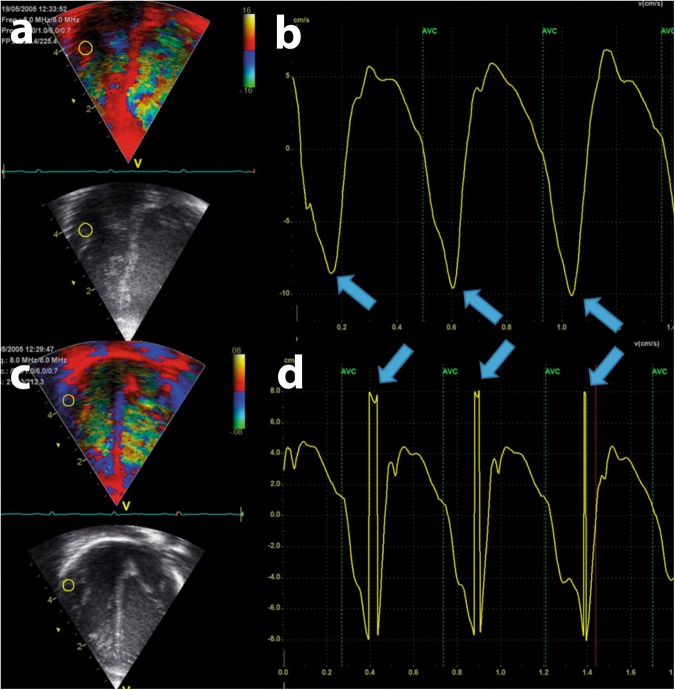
Example of non-aliased and aliased velocity measurements. Both panels show a color tissue Doppler velocity measurements of the right lateral atrioventricular plane in three consecutive heartbeats in a term neonate. The left part of both panels a and c show an apical four-chamber image with a sample area (yellow circle) of 3 × 3 mm. The right panels b and d show the velocity curves. The velocity scale is ±0.16 m/s in the upper panel and ±0.08 m/s in the lower panel. The negative peak velocity in diastole is approximately −0.09 m/s (blue arrows), within the velocity range in the upper panel but outside the velocity range in the lower panel. The ultrasound system therefore shows the velocity correctly in the upper panel and erroneously as a positive velocity close to the upper velocity limit in the lower panel. Note that there is complete fusion of diastolic velocities in the last two heart-cycles in the upper panel; it is not possible to identify two diastolic velocity peaks. *X*-axis: time. *Y*-axis: tissue velocity from the sample area
